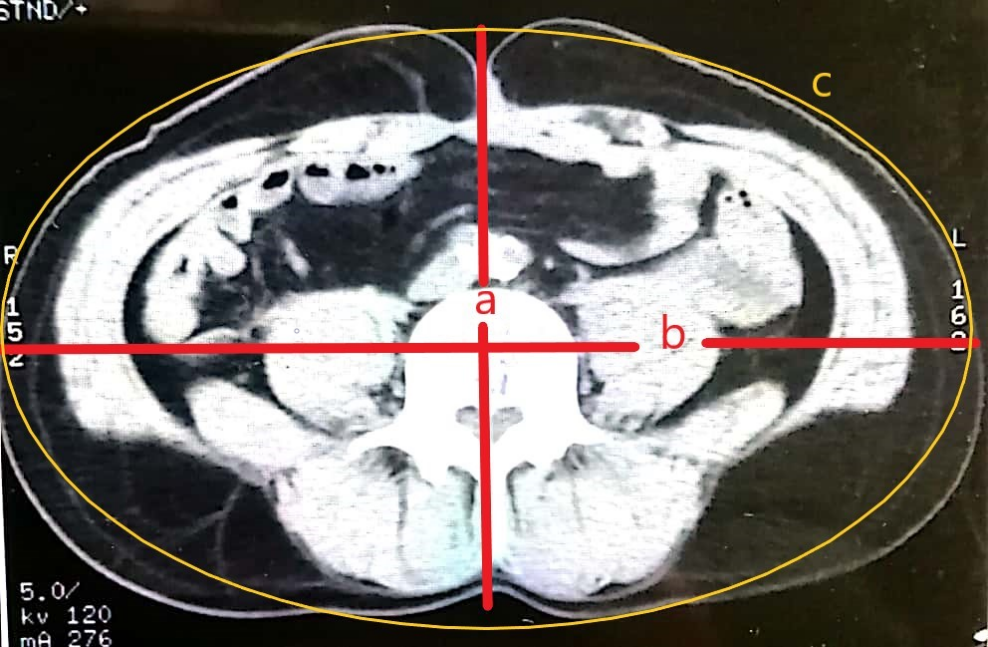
Evaluation of the waist circumference on a computed tomography image using an ellipse perimeter formula. (a) Anterior-posterior diameter, (b) transverse diameter, and (c) ellipse perimeter.
